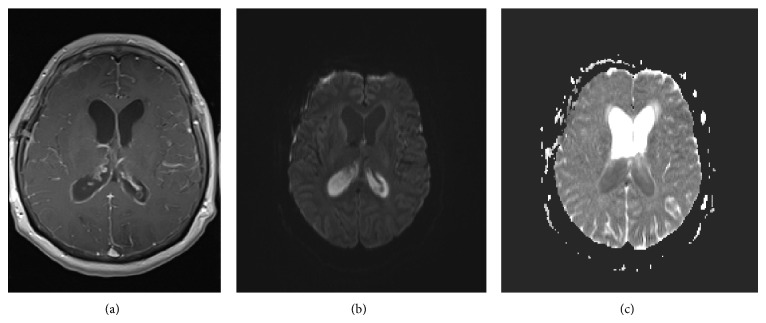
MRI brain. MRI: magnetic resonance imaging. (a) Axial T1 with gadolinium displaying ependymal enhancement in the lateral ventricles. (b) Axial diffusion weighted imaging displaying intraventricular hyperintensity, concerning restriction of ventricular sediment and consistent with ventriculitis/empyema. (c) Apparent diffusion coefficient map confirming intraventricular hypointensity and restricted diffusion of the intraventricular sediment, confirming ventriculitis/empyema.
